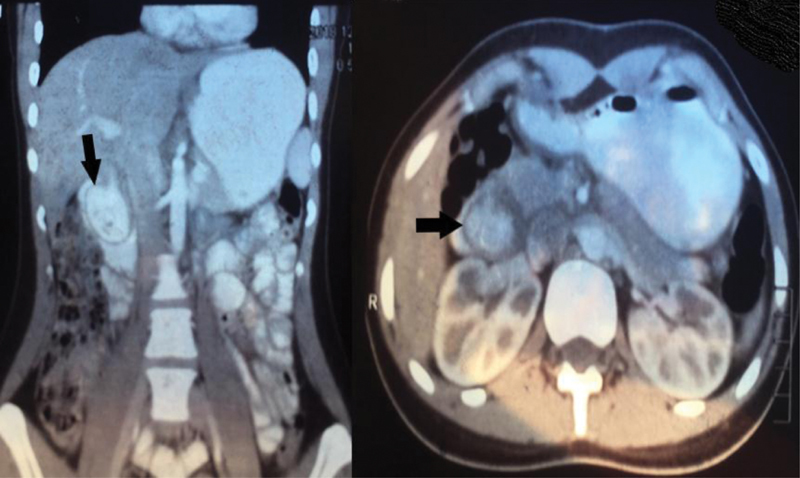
CT scan of the abdomen and pelvis, showing the duodenal diverticulum obstructing the lumen of the duodenum (black arrows). CT, computed tomography.
